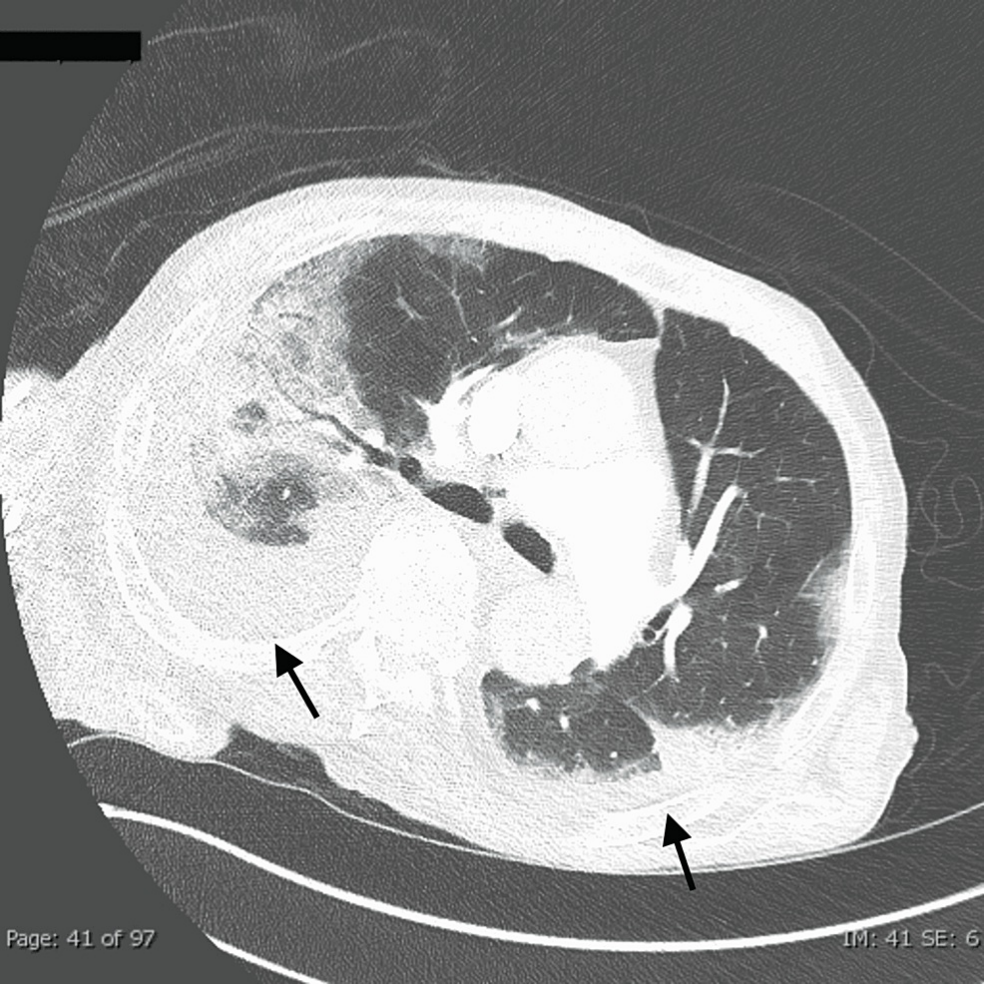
CTPA showing bilateral pulmonary parenchymal opacities and moderate bilateral pleural effusion (arrows) CTPA: computed tomography pulmonary angiogram
